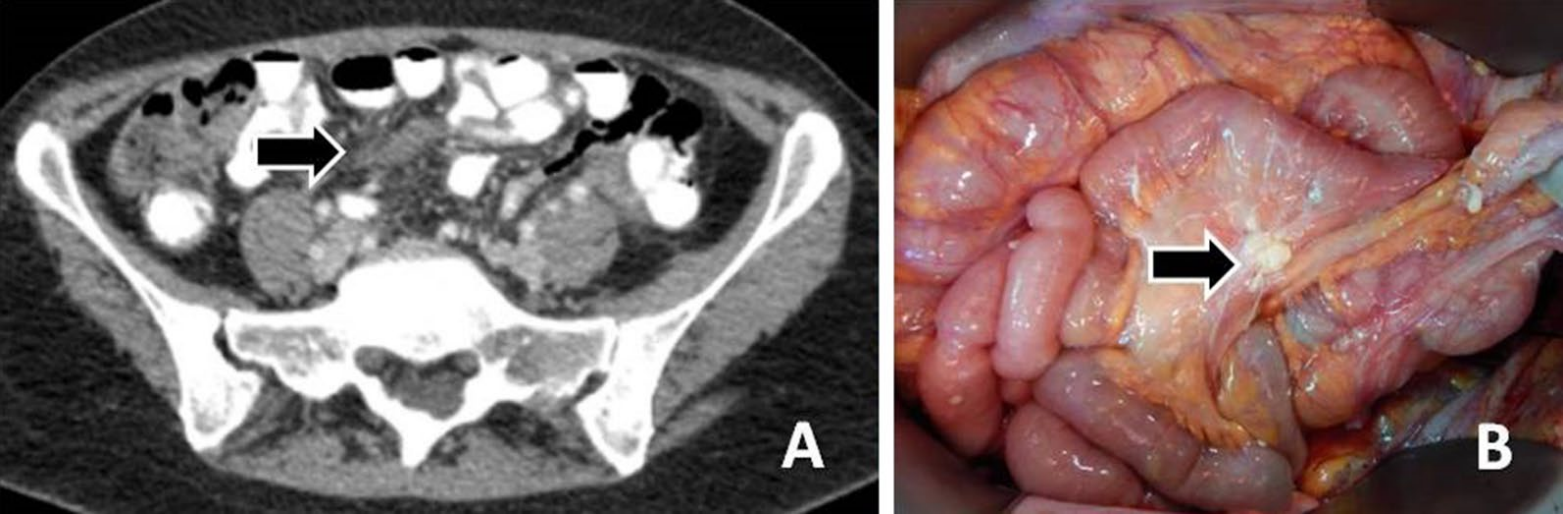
a Contrast-enhanced CT image shows soft tissue density lesion in the mesentery (arrow). b The peritoneal implant confirmed at surgery (arrow)
